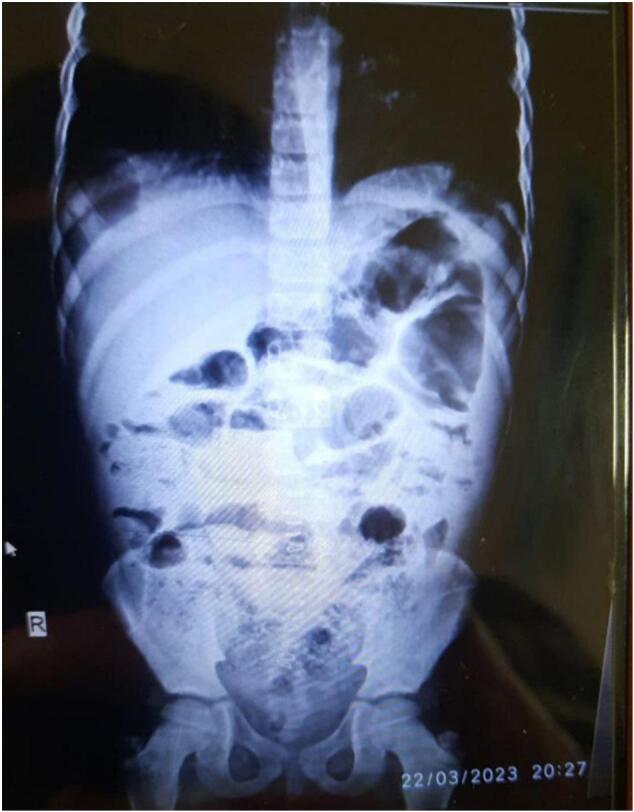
Abdominal X-ray showing multiple dilated small bowel loops with air-fluid levels, consistent with small bowel obstruction.
